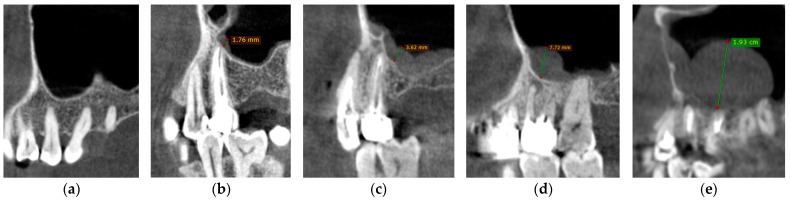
CBCT sagittal reconstructions showing the maxillary sinus mucosa thickness according to the five classes: (a) Class 1; (b) Class 2; (c) Class 3; (d) Class 4; (e) Class 5.
